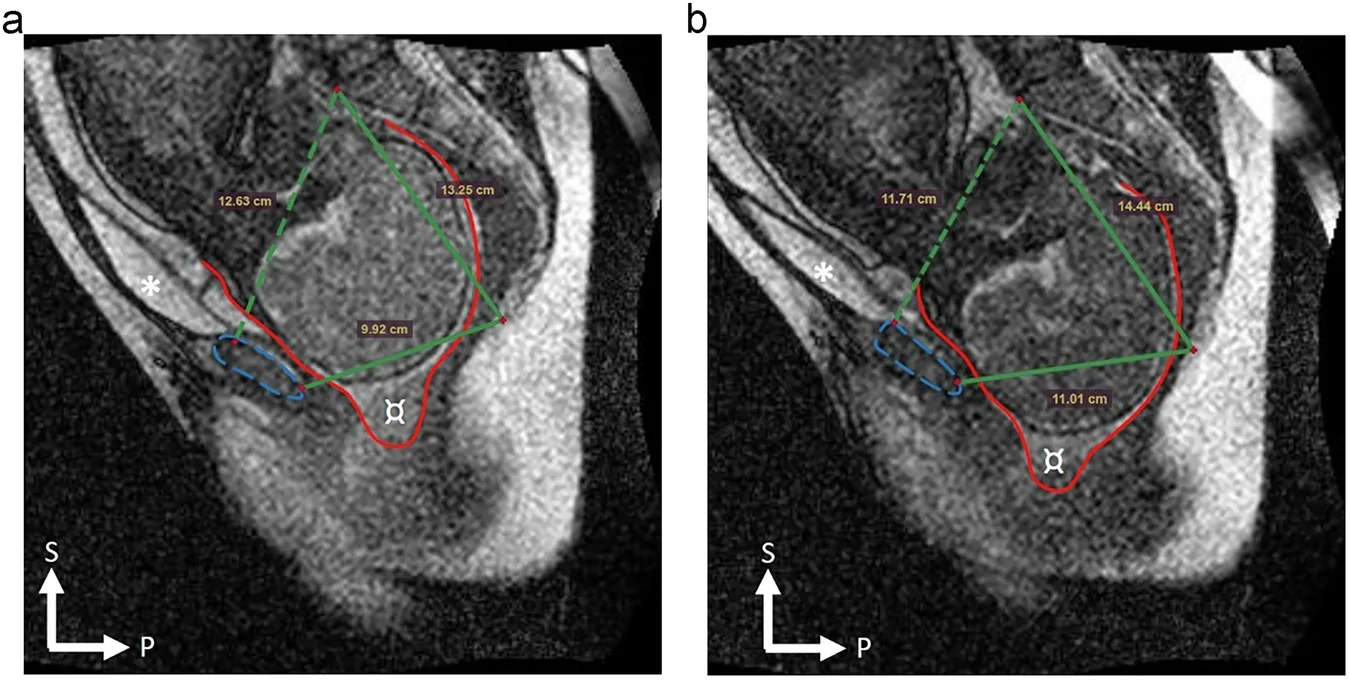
Dynamic sagittal MRI. The fetus (red line) presents himself in an OA position at rest (a) and with maternal expulsive efforts (b). The descent of the fetal head compared to the upper brim plane (green dotted line) is visible. The pubis (blue dotted line) serves as a pivot point for the de-rotation of the fetal head. A subcutaneous hematoma is visible (¤). The hematoma follows the de-rotation of the head around the pubis during expulsive efforts. A partially filled bladder is seen above the level of the pubic bone (*). MRI, Magnetic resonance imaging; OA, Occiput anterior
